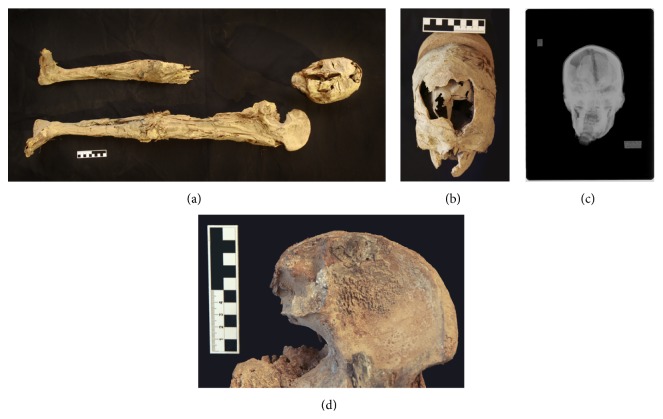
(a), (b), (c), and (d): overview of mummified remains D1 (a), frontal view of head D1 (b), conventional X-ray of head D1 showing among others most likely remnants of the shrunken brain (c), and close-up of alteration in left iliac fossa region of unclear etiology (d).
